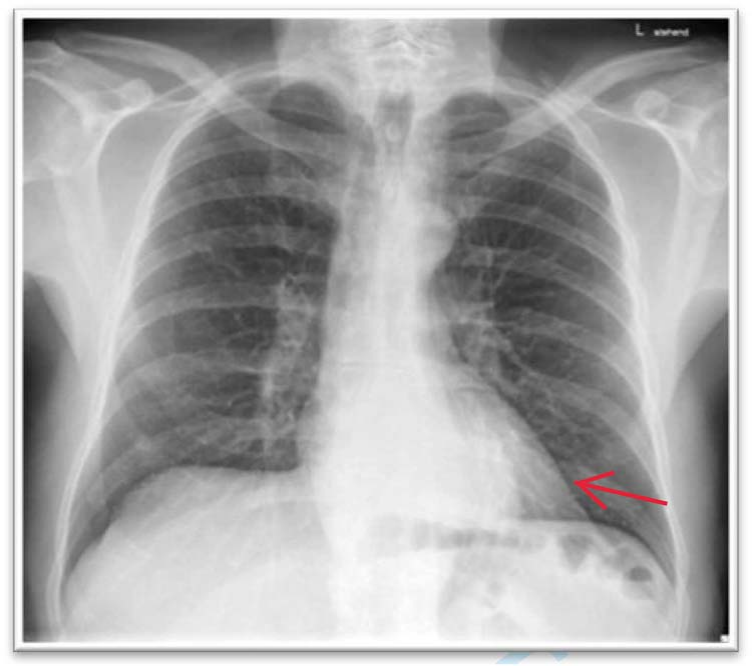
Thoracic X-ray with retrocardiac mass.
